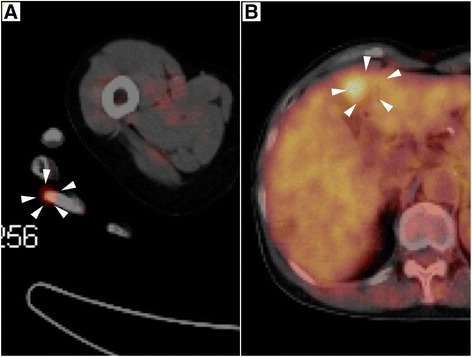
Positron emission tomography/computed tomographic scans. Positron emission tomography/computed tomographic scans showed the uptake of 2-[fluorine-18] fluoro-2-deoxy-D-glucose in the right third finger (white arrowhead, A) and liver segment IV (white arrowhead, B).
